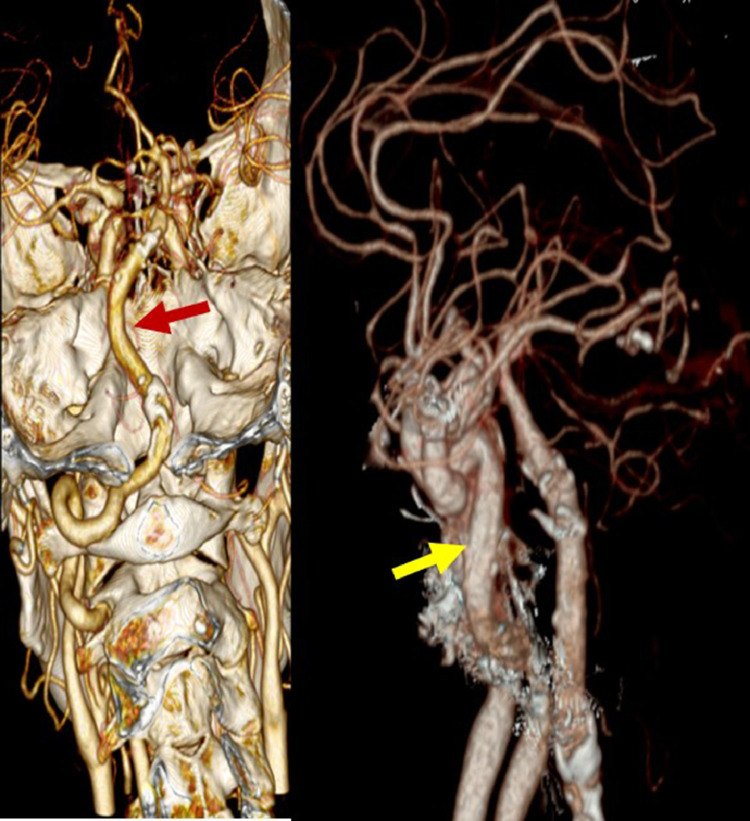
A large vertebrobasilar artery (red arrow) is seen on CT angiography with the same size as the internal carotid artery (yellow arrow). (Color version of figure is available online.)
